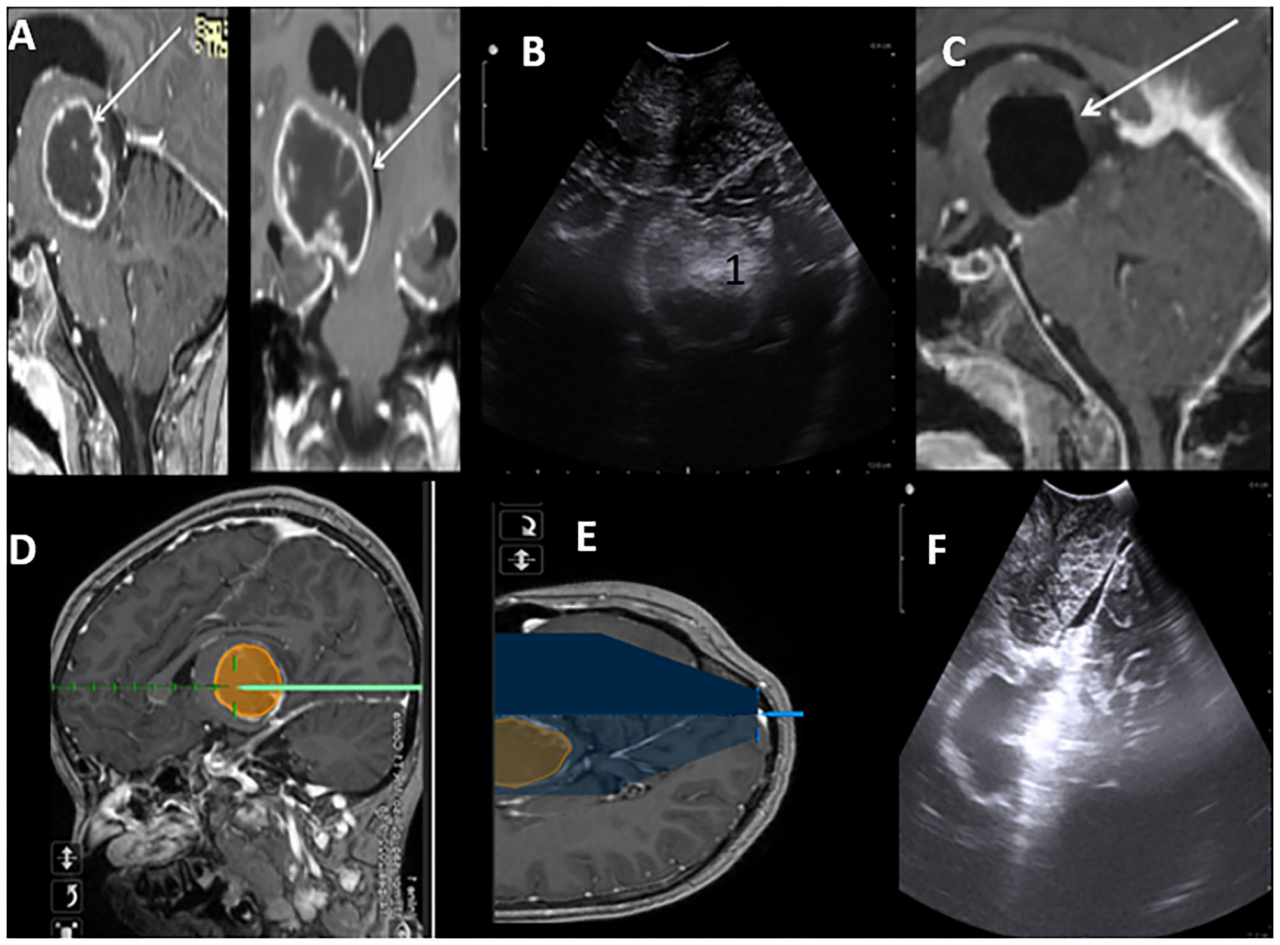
Post Contrast MRI T1WI Sagittal and Coronal sections suggestive of high-grade thalamic glioma (HGG) with cystic central cystic component (A). Brain lab navigation system depicting trajectory to the lesion (D,E). iUS Axil section depicting thalamic hyperechoic lesion (B). Post-resection iUS Sagital section depicting wall of the cavity after complete resection (F). MRI in concordance with ius showing gross total resection of the HGG (C).
